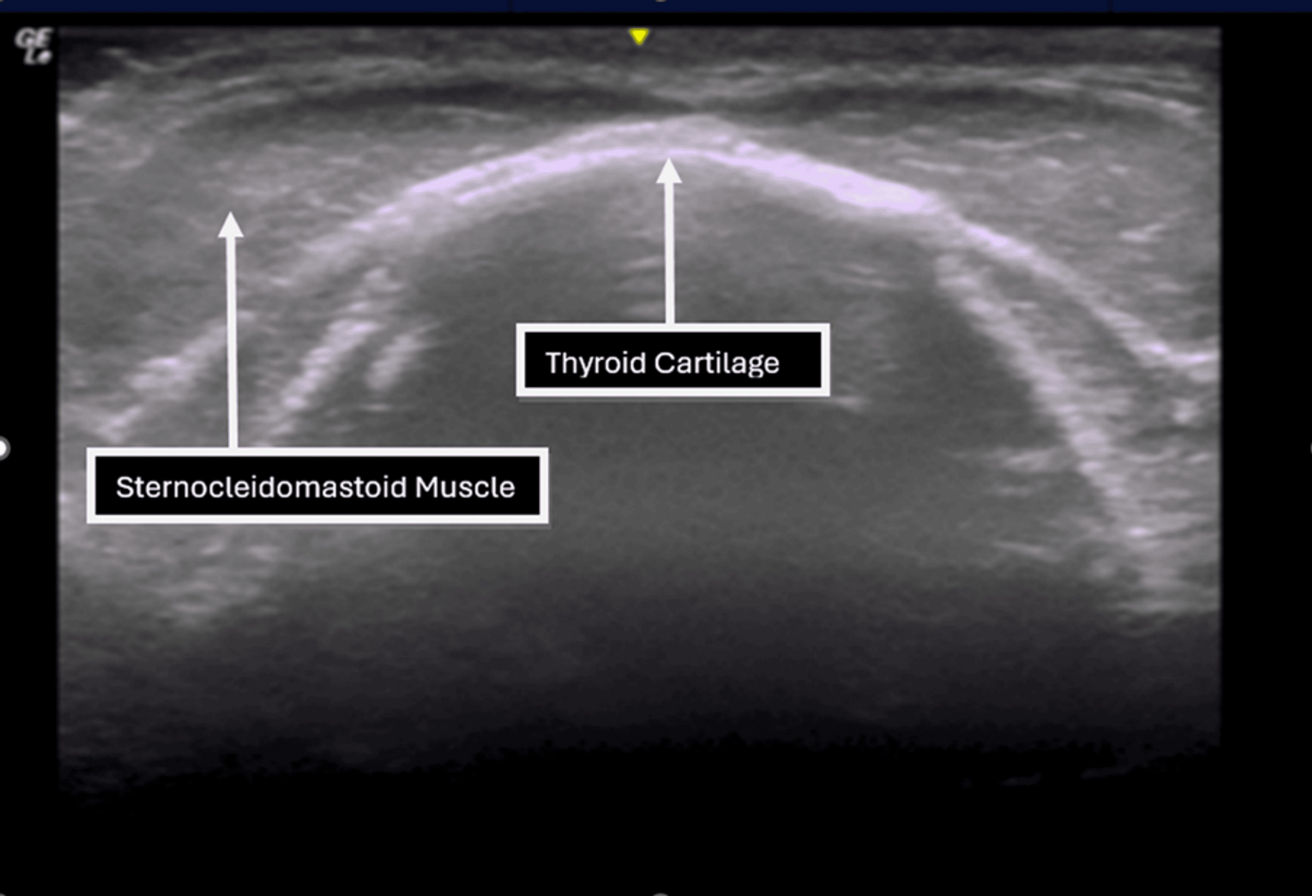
Transverse ultrasound image at the level of the inferior border of the thyroid cartilage.
